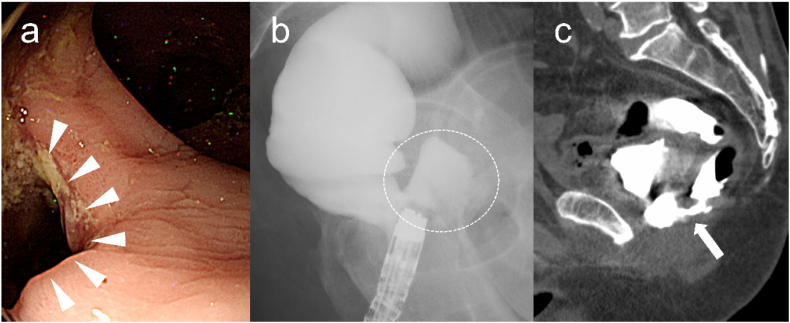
Examination findings of the patient who complained of fecal discharge from the urethra after robot-assisted laparoscopic radical prostatectomy. a) Colonoscopy: A large perforation of 2 cm in size (arrowhead) was found in the anterior wall of the lower rectum. b) Enterography: Leakage and accumulation of contrast medium on the ventral side of the rectum (dotted circle). c) CT: Contrast media traffic between the rectum and the urethra (arrow).
